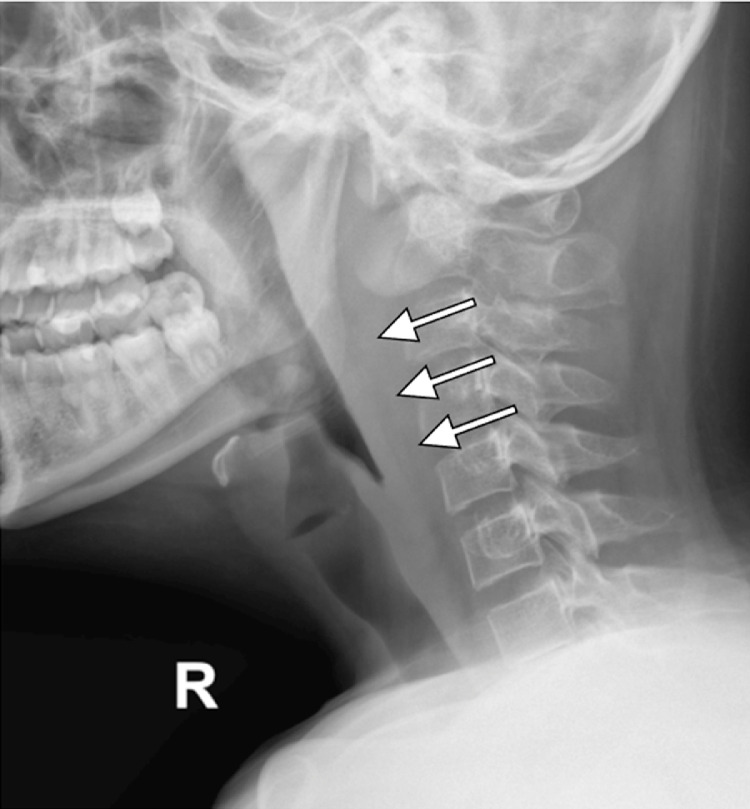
Lateral cervical radiography showing thickening of retropharyngeal and prevertebral soft tissues at the level of the craniocervical junction to the C5 vertebrae (white arrows).
